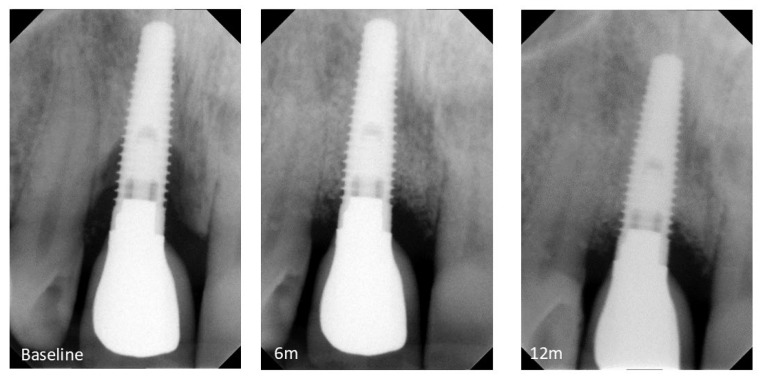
Radiographic follow-up of the reconstructive surgical protocol over a 12-month period (6m: 6 months; 12m: 12 months).
